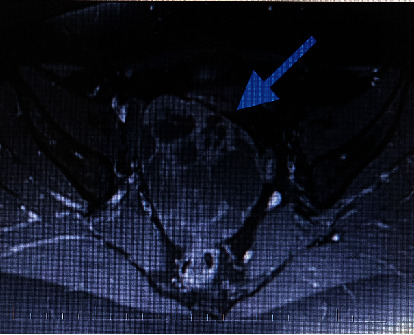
Magnetic resonance imaging, axial view: a solid-cystic tumour in the lesser pelvis with a significant solid component. The blue arrow is pointing to the pelvic tumour.
